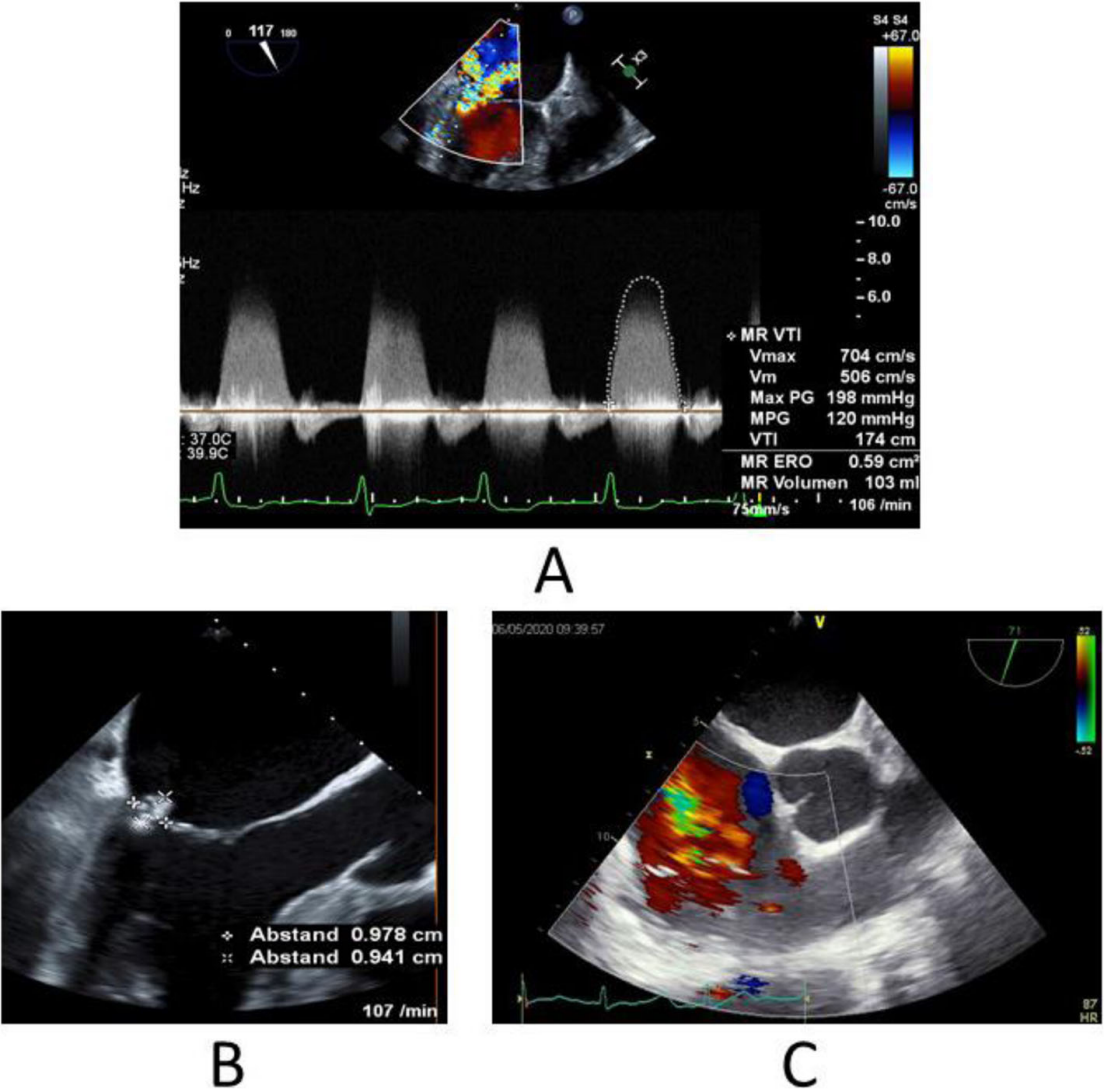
Preoperative Echocardiography. a Mitral valve regurgitation; b 10 × 12 mm vegetation of the mitral valve; c tricuspid valve regurgitation
